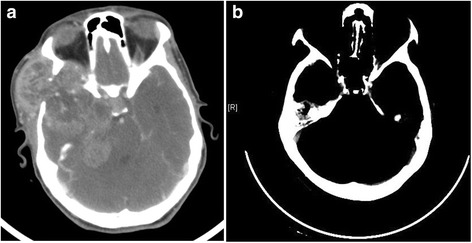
a CT head axial image (patient # 1) showing a destructive recurrent mass in the right sphenoid wing, extending into middle and posterior cranial fossae with destruction of a large portion of the skull base; b CT head (patient # 4) demonstrating heterogeneous soft tissue recurrent mass involving the right mastoid air cells, mastoid bone and occipital bone associated with bone destruction
